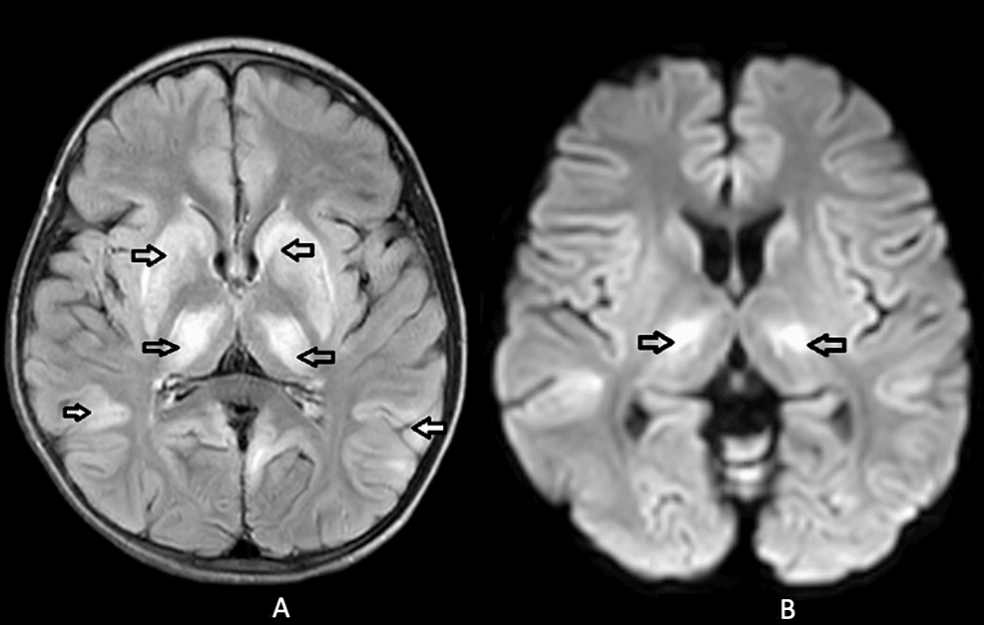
(A) T2 FLAIR hyperintensities (arrows) in the bilateral basal ganglia with cortical and subcortical hyperintensities in bilateral parietal lobes. (B) Diffused restriction in the bilateral basal ganglia suggestive of atypical Wilson disease (arrows) FLAIR, fluid-attenuated inversion recovery
